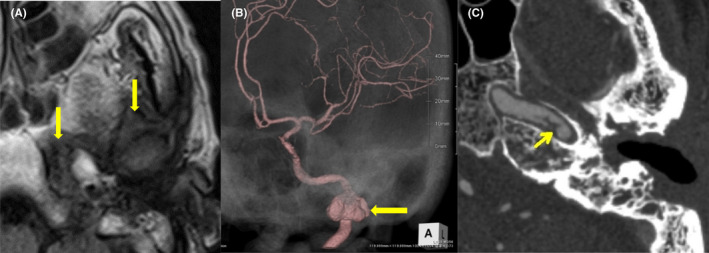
(A) Magnetic resonance imaging (T2‐weighted image) showed low intensity (yellow arrow) extending from the left external auditory canal to the pyramidal bone, temporomandibular joint fossa, and masticator space. (B) Magnetic resonance angiography revealed irregular dilatation of the left internal carotid artery. (C) Computed tomography angiography indicated an irregular mass in the left internal carotid artery
